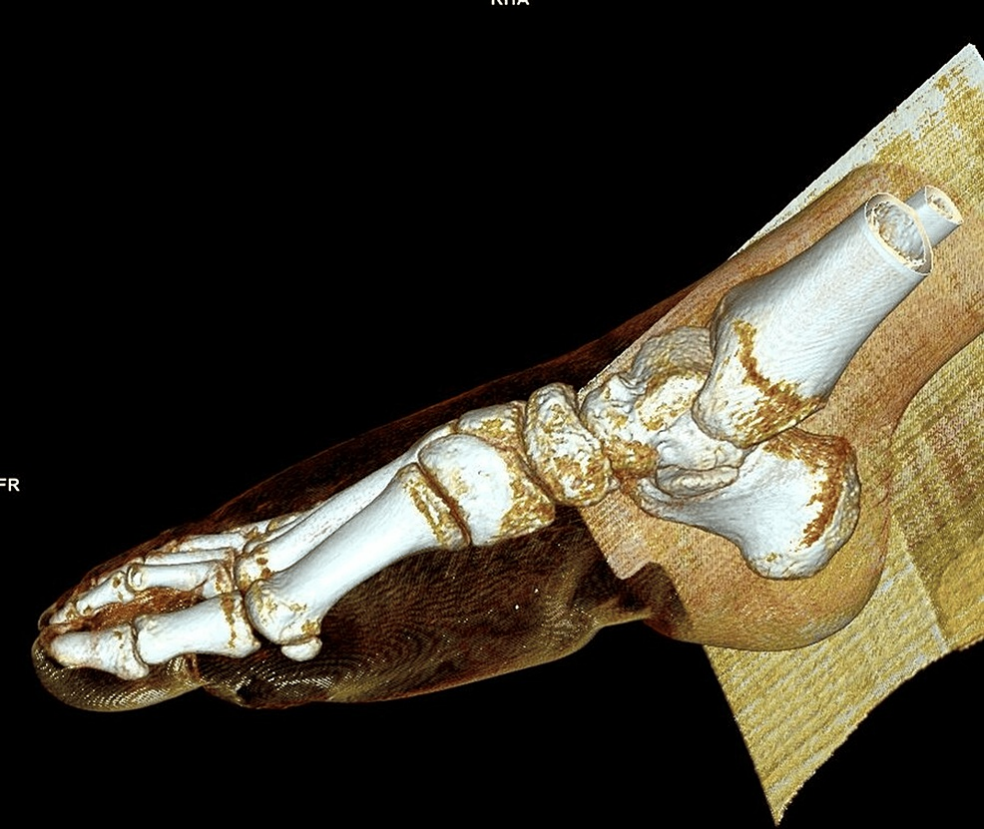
Non-contrast CT (NCCT) sagittal reconstruction of the bone and soft tissue
